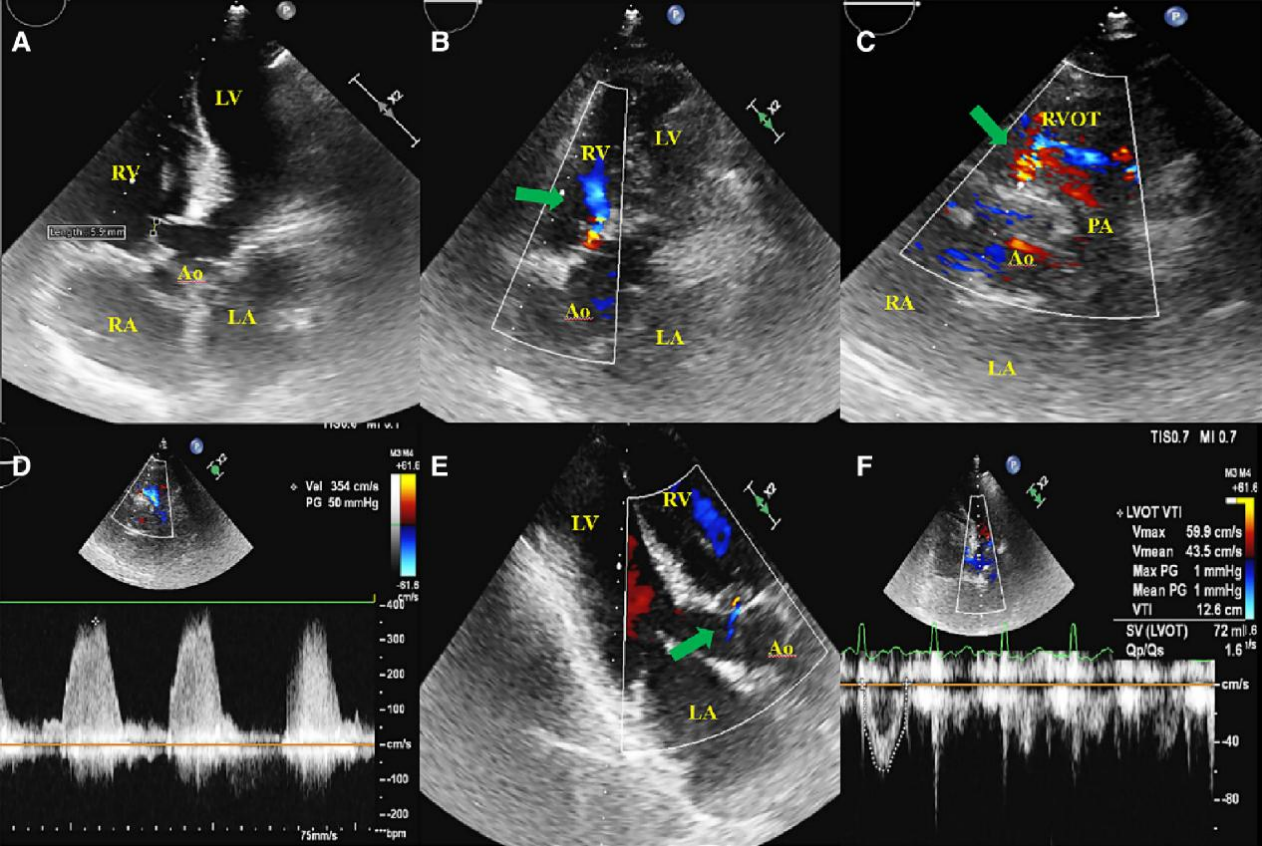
(*A*) Apical 5-chamber view showing a small restrictive perimembranous ventricular septal defect (5.5 mm) with predominantly (*B*) left-to-right shunting (arrow) on modified apical 5-chamber view. (*C*) Parasternal short-axis level of great vessels confirming the communication (arrow) with a (*D*) maximum gradient of 50 mmHg. (*E*) Parasternal long axis view with proof of right-to-left shunting (arrow). (*F*) Calculation of pulmonary-to-systemic blood flow ratio—1.6. A5C, apical 5 chamber; Ao, aorta; LA, left atrium; LV, left ventricle; PA, pulmonary artery; PLAX, parasternal long axis; PSAX, parasternal short axis; RA, right atrium; RV, right ventricle; RVOT, right ventricular outflow tract; VSD, ventricular septal defect.
